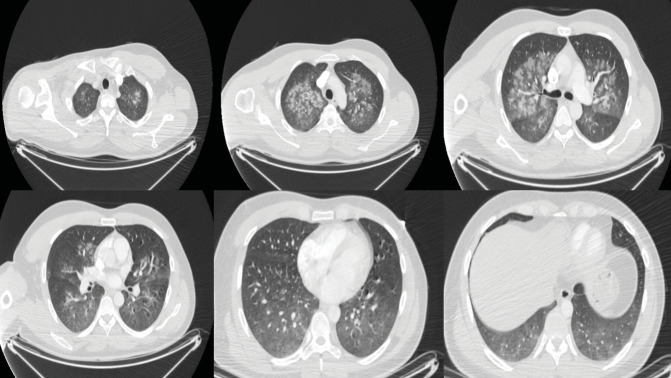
Figure 2. Axiel CT images show bilateral diffuse
